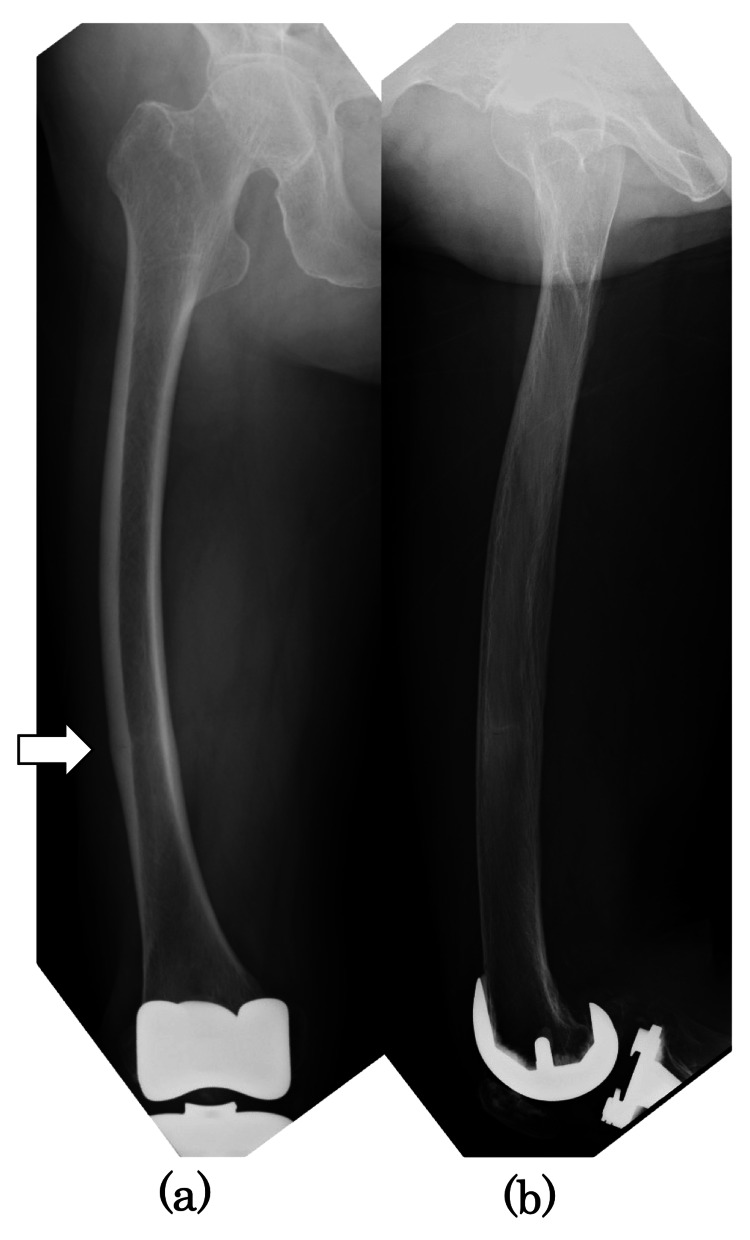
Preoperative radiographs Preoperative radiographs: (a) anteroposterior and (b) lateral images. The white arrow showed a thickening of the lateral cortical bone in the diaphysis.
